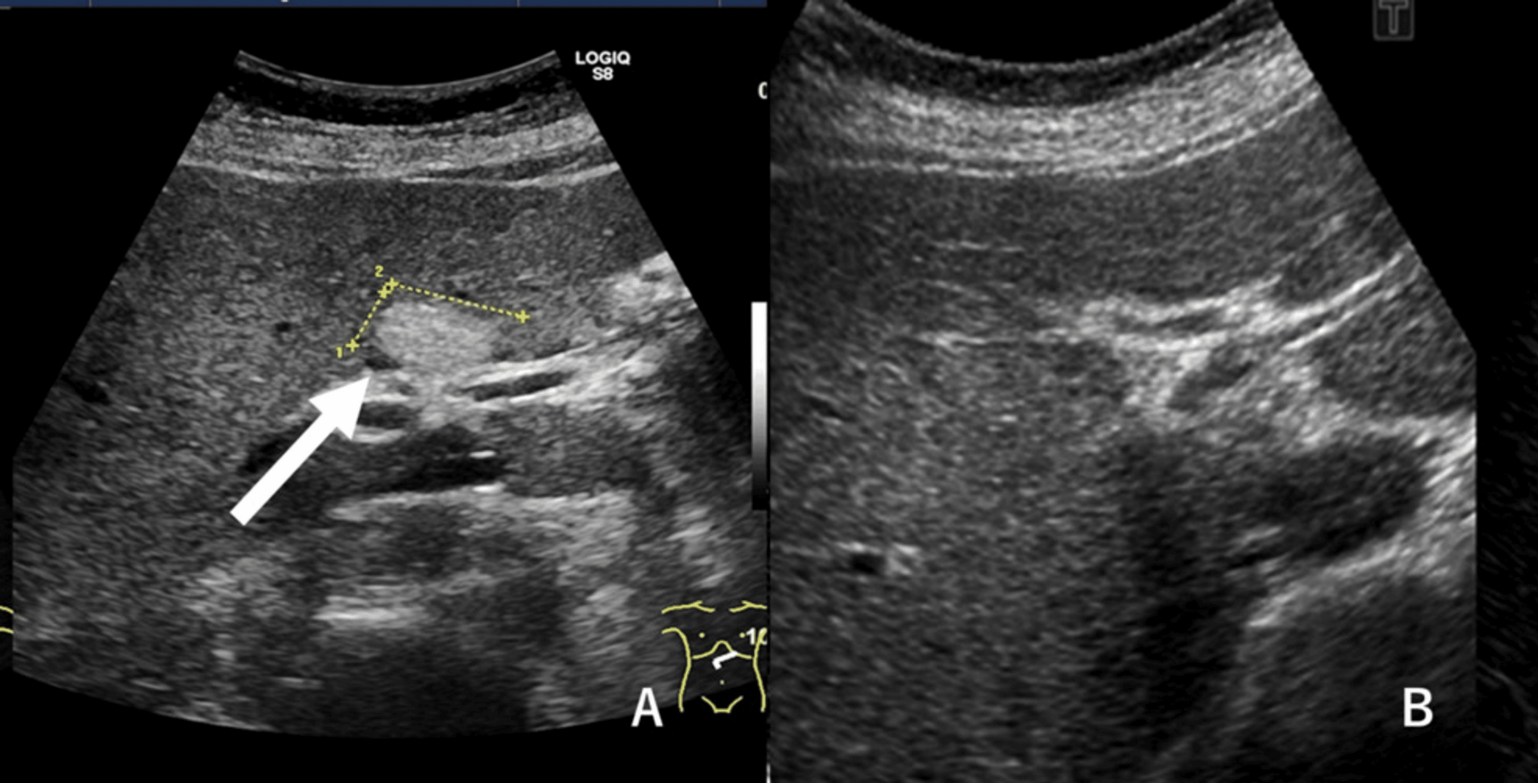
Abdominal ultrasonography findings in segment IV (S4) A: baseline ultrasonography shows a hyperechoic lesion (approximately 25 mm) in segment IV (S4) near the hepatic hilum (arrow); B: follow-up ultrasonography three months after intensive glycemic control shows complete disappearance of the lesion. S4: segment IV; US: ultrasonography
